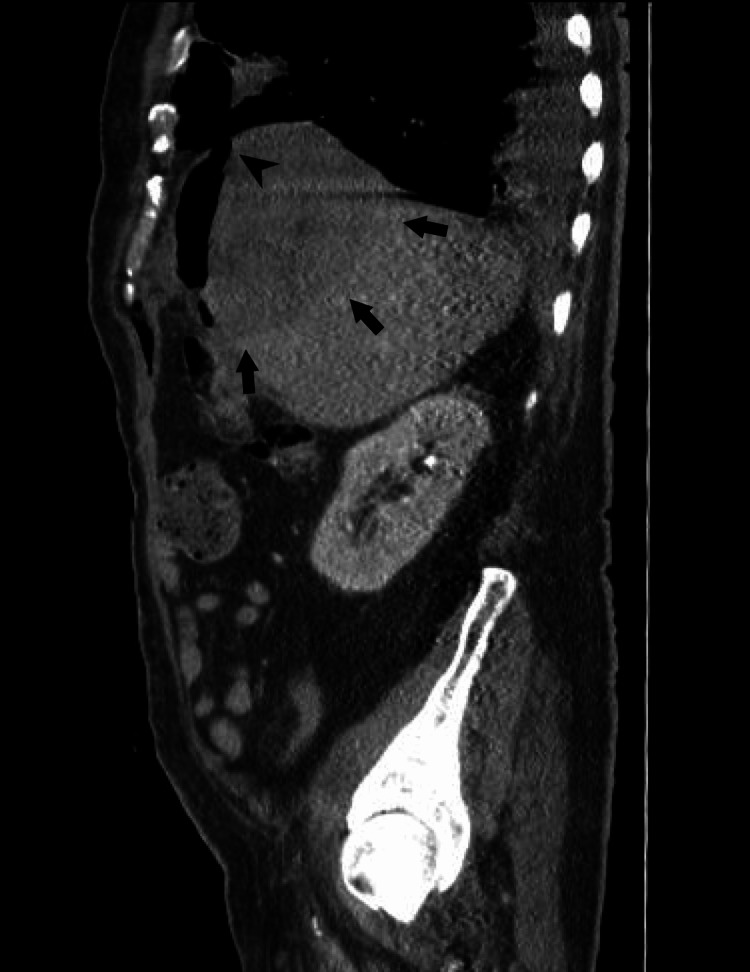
Sagittal CT scan of the patient's abdomen. The image shows a sagittal view of the liver abscess after percutaneous drainage, marked by arrows. The arrowhead indicates the diaphragmatic perforation.
